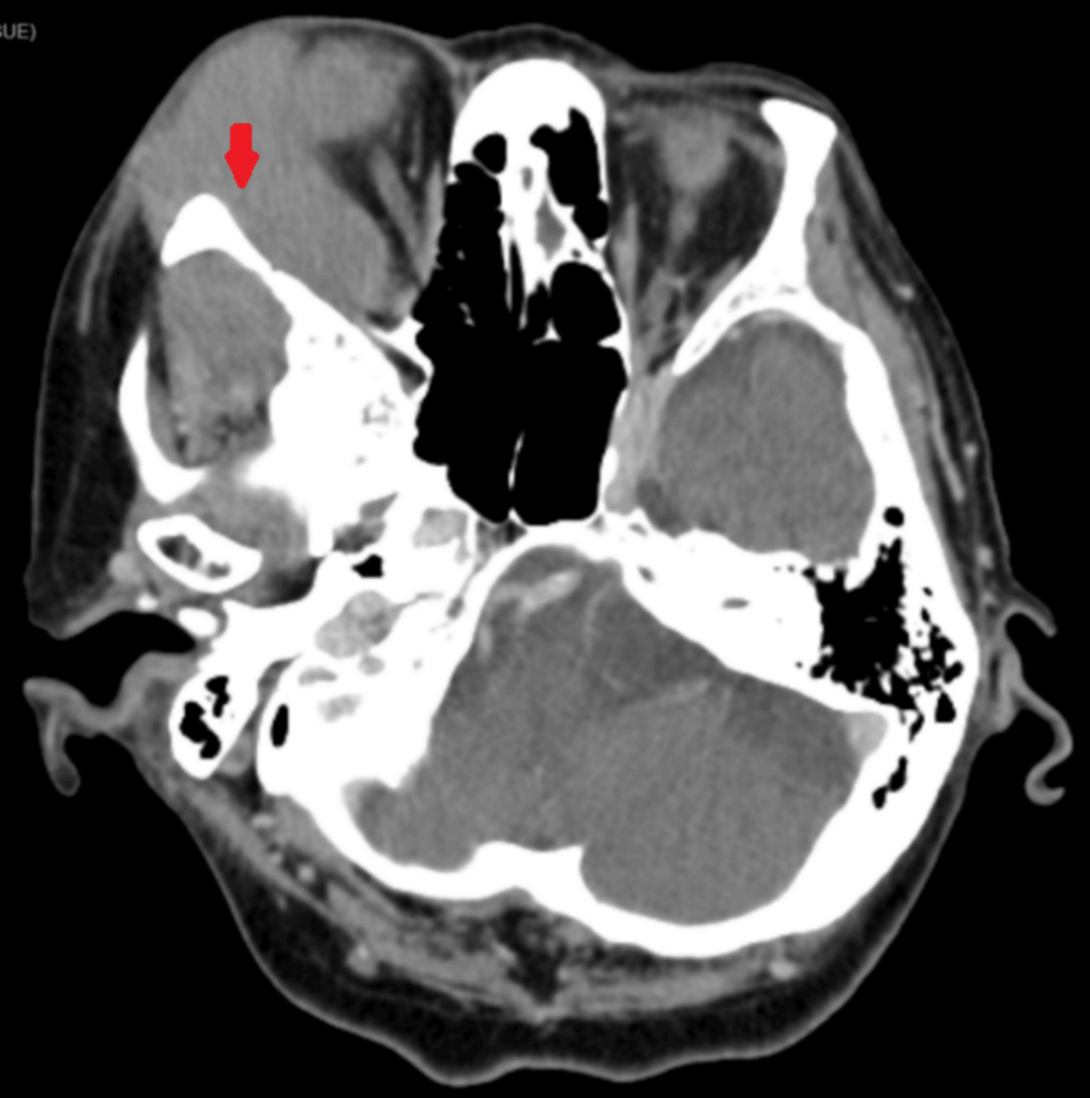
CT brain and orbit (axial view) on the first presentation CT: computed tomography
